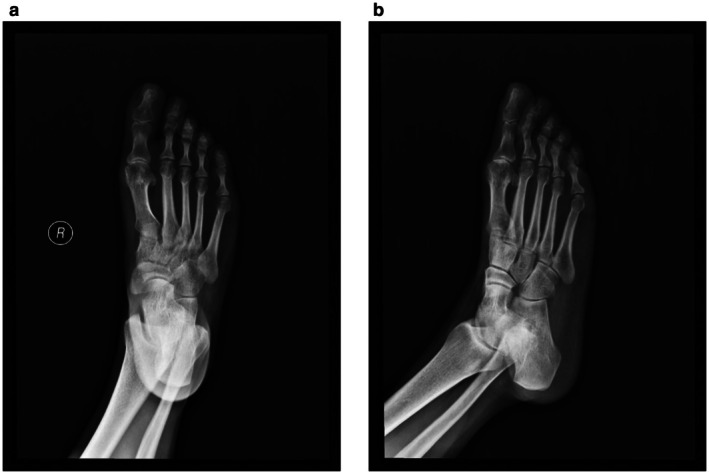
Radiographic imaging of the right foot obtained 8 weeks following removal of the Kirschner wires confirms complete fracture healing.
